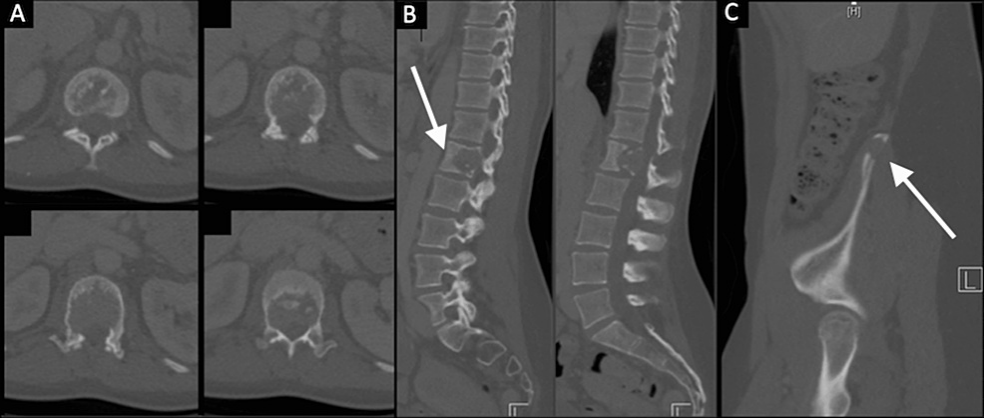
Preoperative computed tomography scan of the lumbosacral spine and pelvis. A: axial views showing a significant amount of lysis to the vertebral body and both pedicles of L1 with some volume of the epidural disease. B: sagittal cuts demonstrating the extent of the involvement of the anterior column and early signs of pathological fracture to both superior and inferior endplates. C: small lytic lesion reported as likely to represent a metastasis with the right iliac crest. This was felt to be accessible for stereotactic radiotherapy and treated subsequently.
